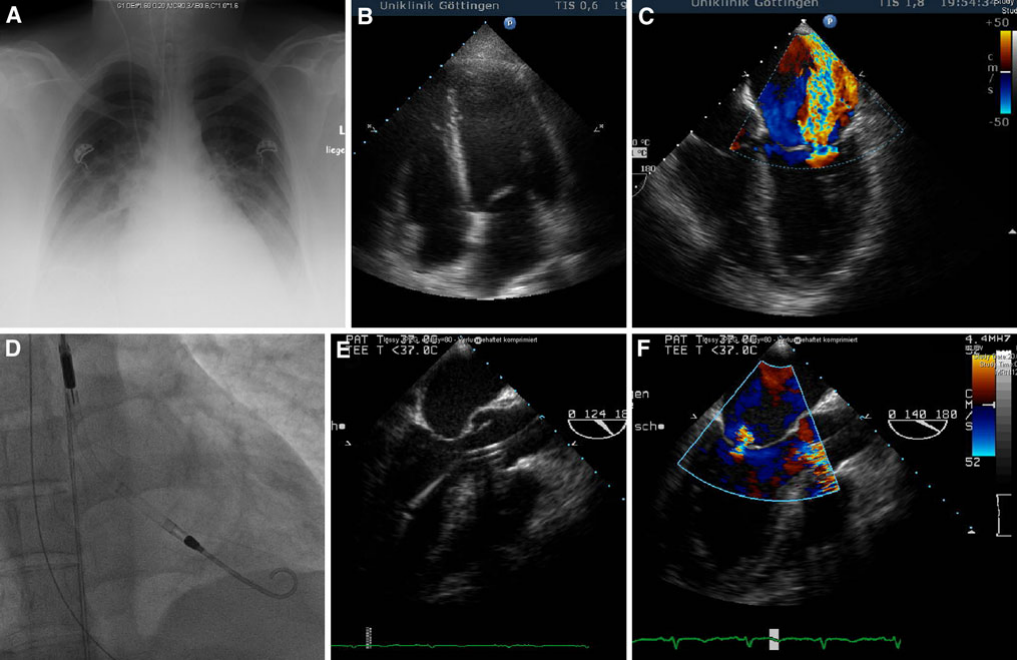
The patient with PPCM presented with bilateral infiltrates and pleural effusions, as shown in the thoracic X-ray (a). The transthoracic echocardiogram shows a dilated left ventricle (apical 4 chamber view; b) and the transesophageal echocardiogram (TEE) indicates an acute severe functional mitral regurgitation (ME 4 chamber view; c). An Impella LP 2.5 percutaneous micro-axial pump assist device was implanted under fluoroscopic control (d) and its correct position through the aortic valve could be verified by TEE (ME long axis view; e). After Impella pump implantation a reduction of mitral regurgitation could be shown (f). Philips SONOS 5500 with IPx-1 and HD11XE with S7-2 were used
